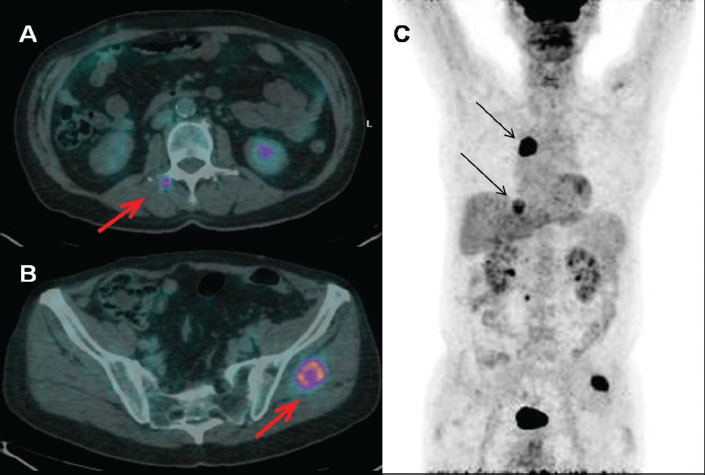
Increased uptake of the radiopharmaceutical in muscle and pulmonary metastases (FDG-PET/CT). A: right para-vertebral muscle close to the soma of L2 (fused transaxial images: red arrow); B: left gluteus muscle (fused transaxial images: red arrow); C: pulmonary lesions at right upper lobe and at the ipsilateral base (maximum intensity projection image: black arrows).
